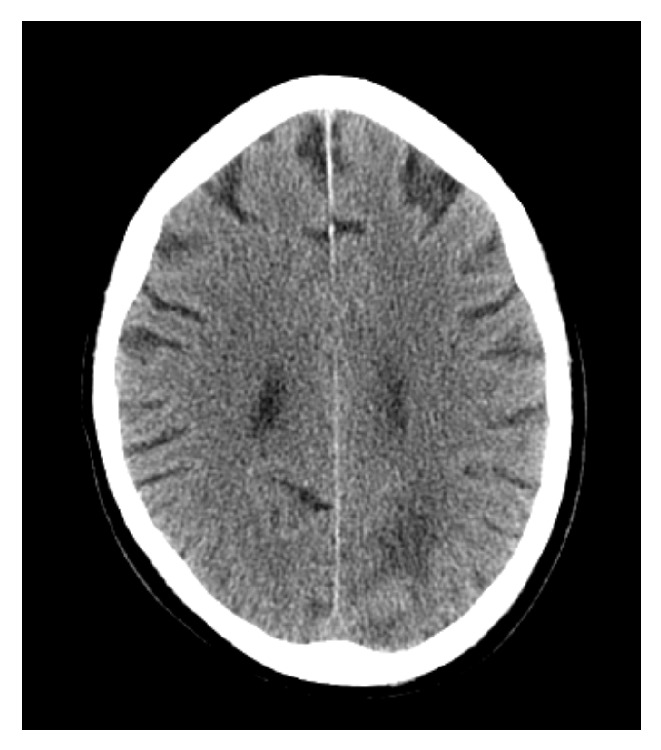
Head CT scan showing multiple brain metastases left breast tissue (low power field).
